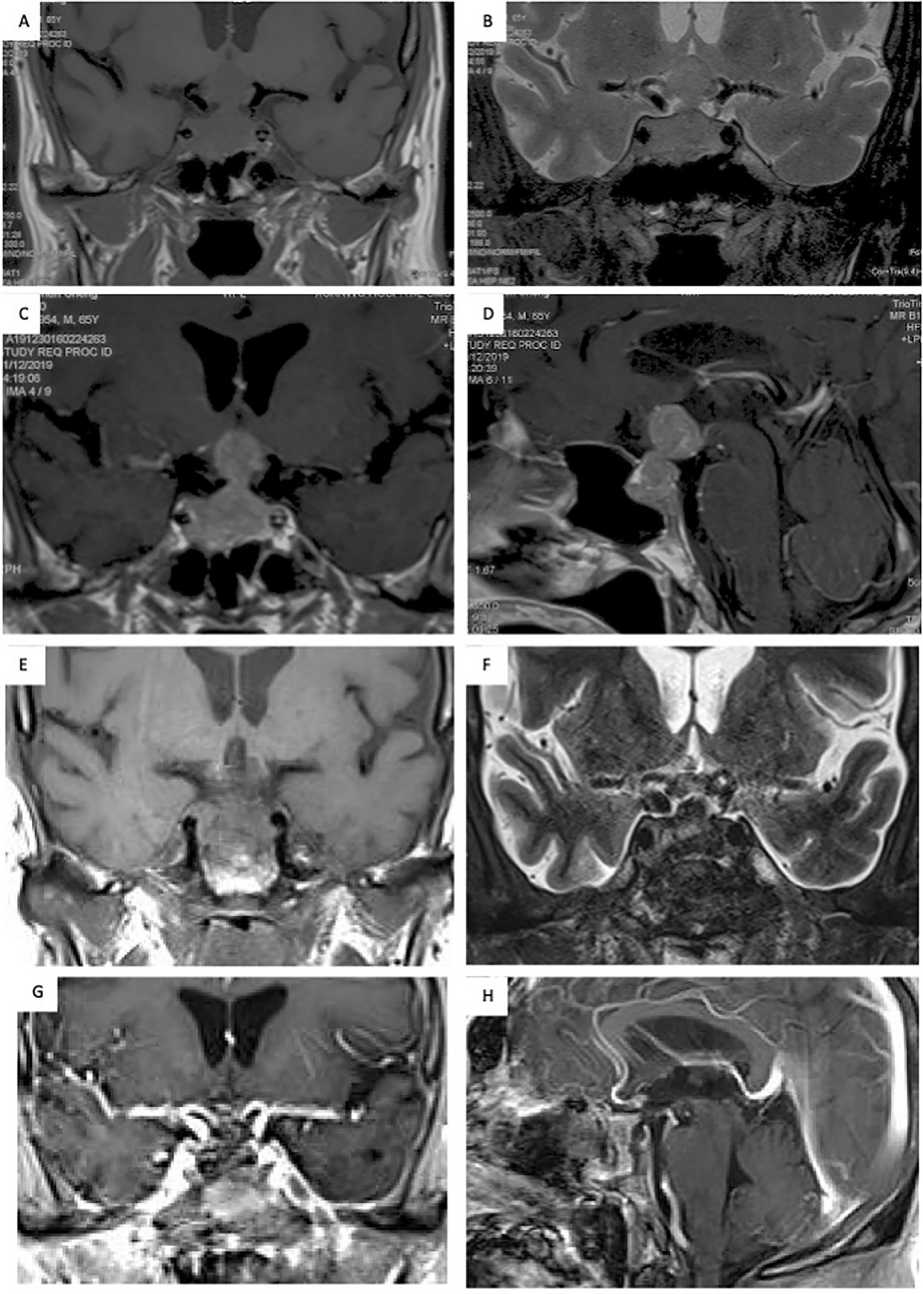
Seller MRI scans showed a sellar lesion with suprasellar extension and compression of the optic chiasm. (A, B) The lesion located in the sellar region presented with an isointense signal on T1- and T2-weighted MRI; (C, D) The mass was uniformly enhanced on MRI after contrast enhancement, and a pituitary adenoma was highly suspected. (E–H) The postoperative MRI showed total resection of the lesion.
